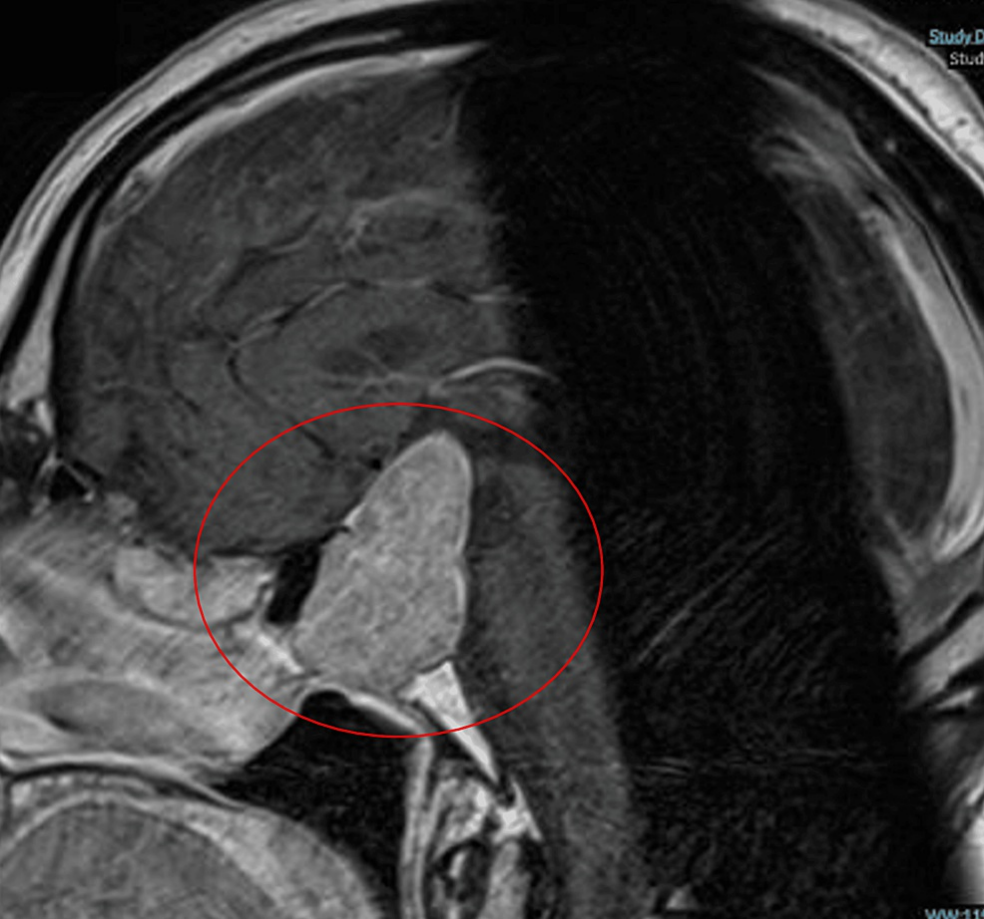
MRI brain showing a large pituitary macroadenoma
